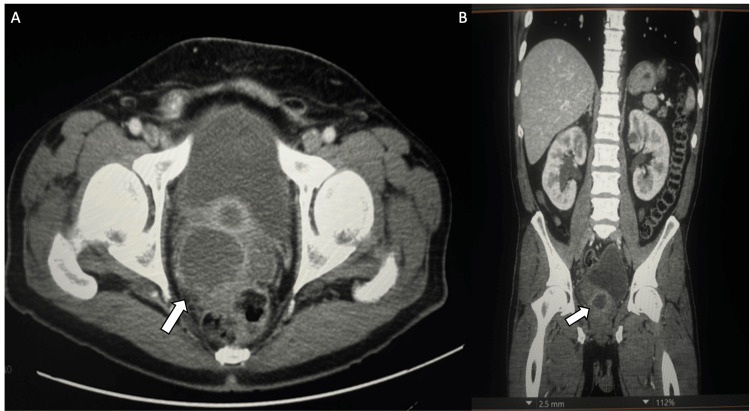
CT abdomen/pelvis with contrast revealed a 6 cm multiloculated prostatic abscess (arrow) A: axial view; B: coronal view
